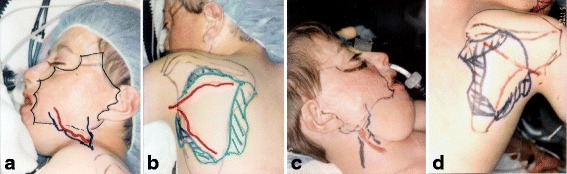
Case 1. a Keloid excision LT cheek unit. Doppler auscultation of facial vessels. b Design of pre-patterned scapular flap. *Hash marks* refer to intraoperative sculpting. Doppler auscultation of superficial circumflex scapular vessels. c Keloid excision RT cheek unit. d Design of pre-patterned scapular flap. (Reprinted from Rose EH. Pre-patterned, sculpted free flaps for facial burns. In: : Hyakusoku H, Orgill DP, Teot L, Pribaz JJ, Ogawa R (eds). *Color Atlas of Burn Surgery*. Heidelberg: Springer; 2010)
